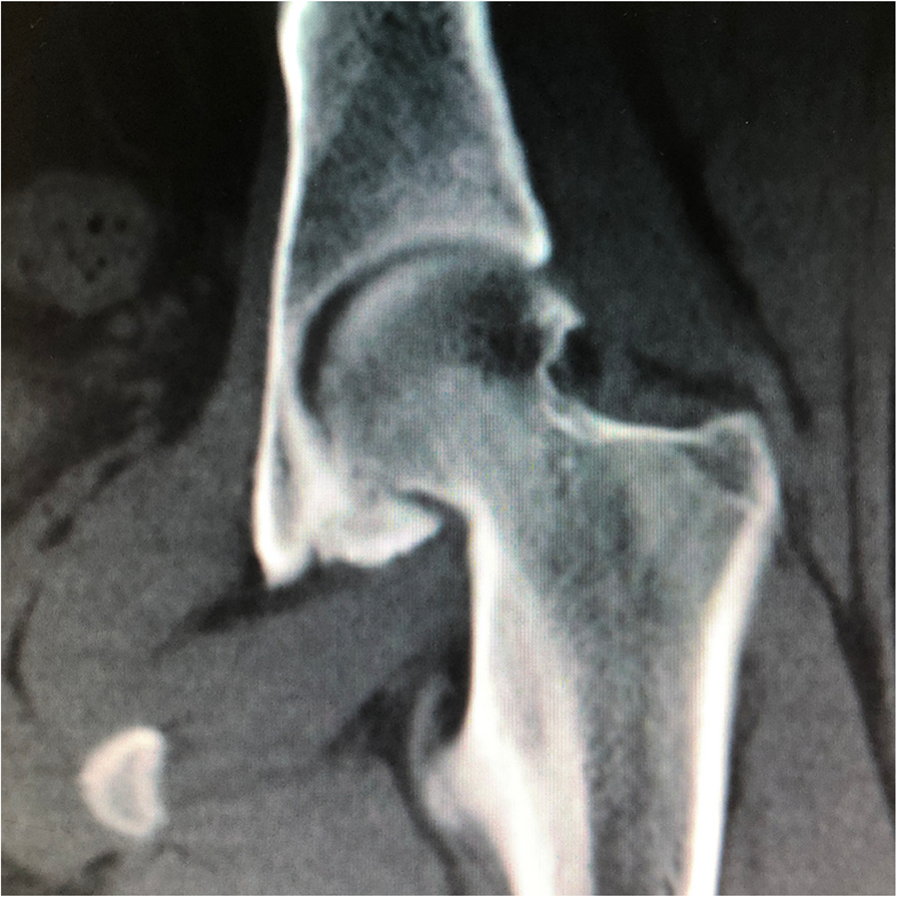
Coronal CT showing OO and a periosteal reaction in the joint surface of the acetabulum
